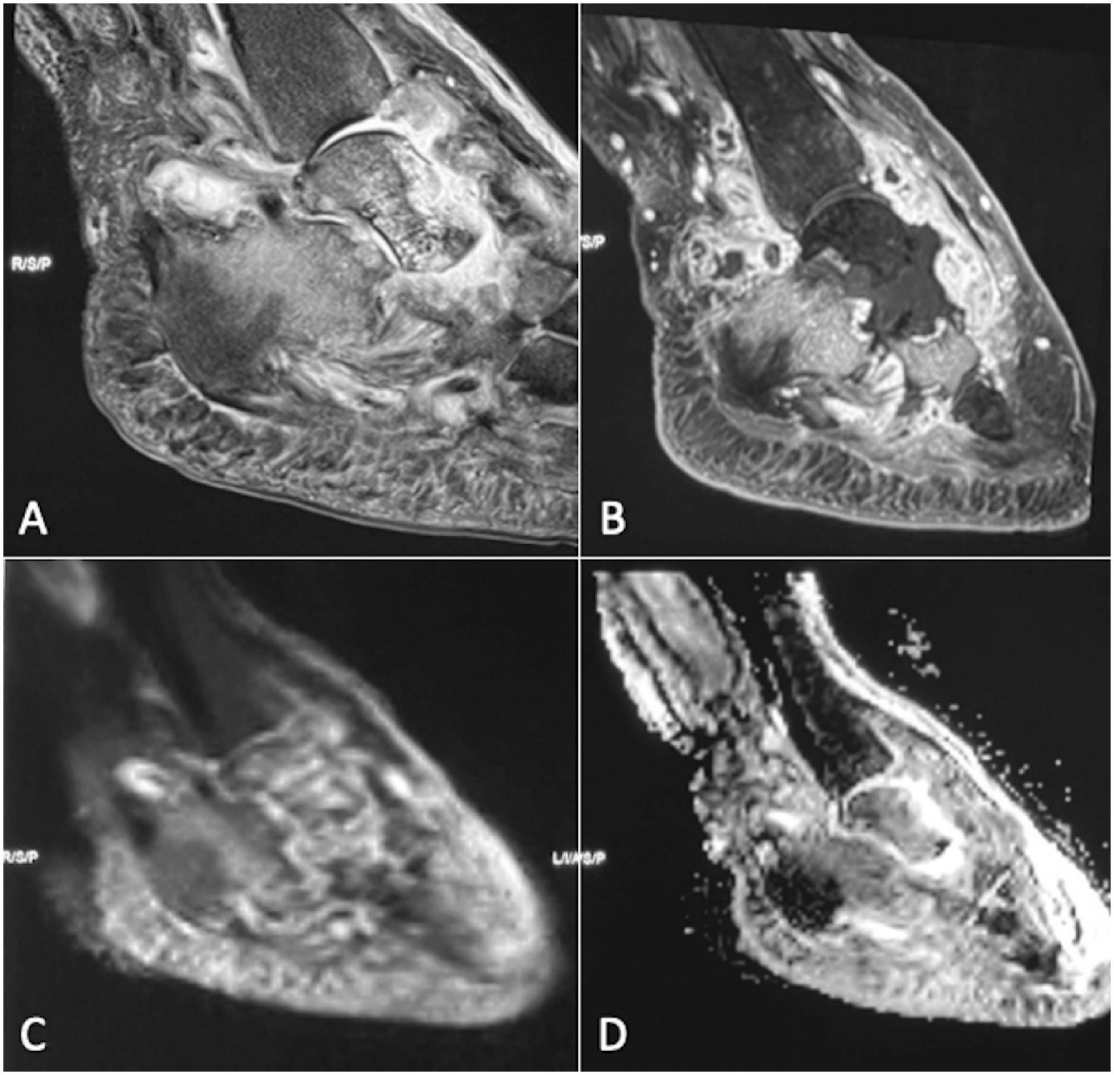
A 54-year-old female presents with a diabetic foot. The PDFS sagittal image (A) reveals involvement of the talus bone and adjacent joints, along with marrow oedema and fluid collections consistent with osteomyelitis. The contrast-enhanced MRI (B) demonstrates enhancement around the periphery of the collection, with non-enhancing necrotic areas within talus with bone erosions of talus, posterior superior calcaneus, cuboid and naviculum. DWI (C) shows hyperintense regions, accompanied by increased signal on the ADC map (D), with ghost sign consistent with proven osteomyelitis. These findings are unlike Charcot neuroarthropathy, which typically shows subchondral bone fractures, disorganization and subchondral cysts.
